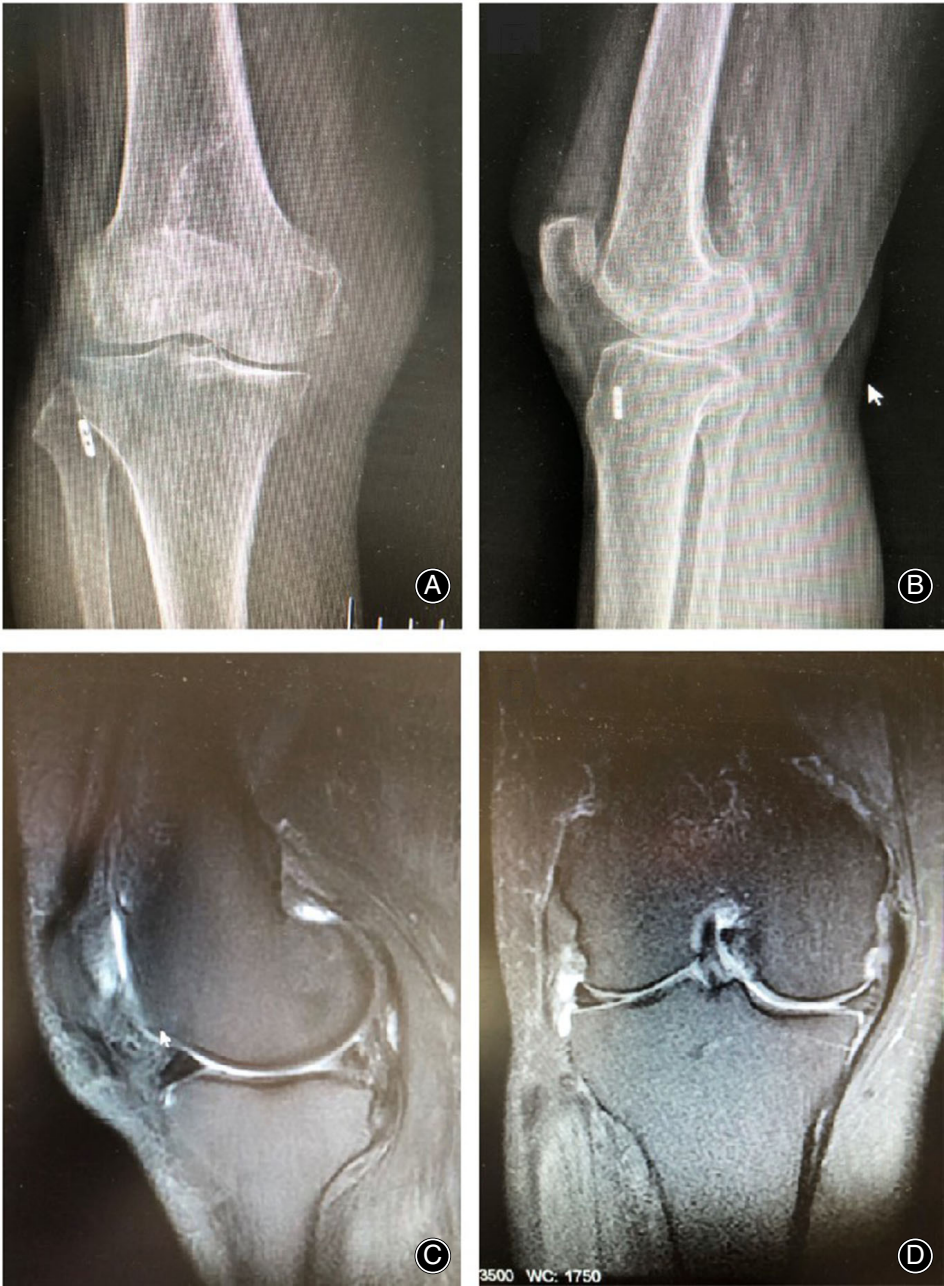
X‐rays and magnetic resonance imaging (MRI) of the affected knee joint at 7 months follow‐up. (A, B) X‐rays of the knee joint showed good positioning of the micro titanium plate at the external mouth of the tibial tunnel. (C, D) MRI showed that the deficit in the posterior root of the medial meniscus was filled and occupied by the medial meniscus stump reconstructed by the suture anchor (C), without an increase in protrusion deformity (D).
